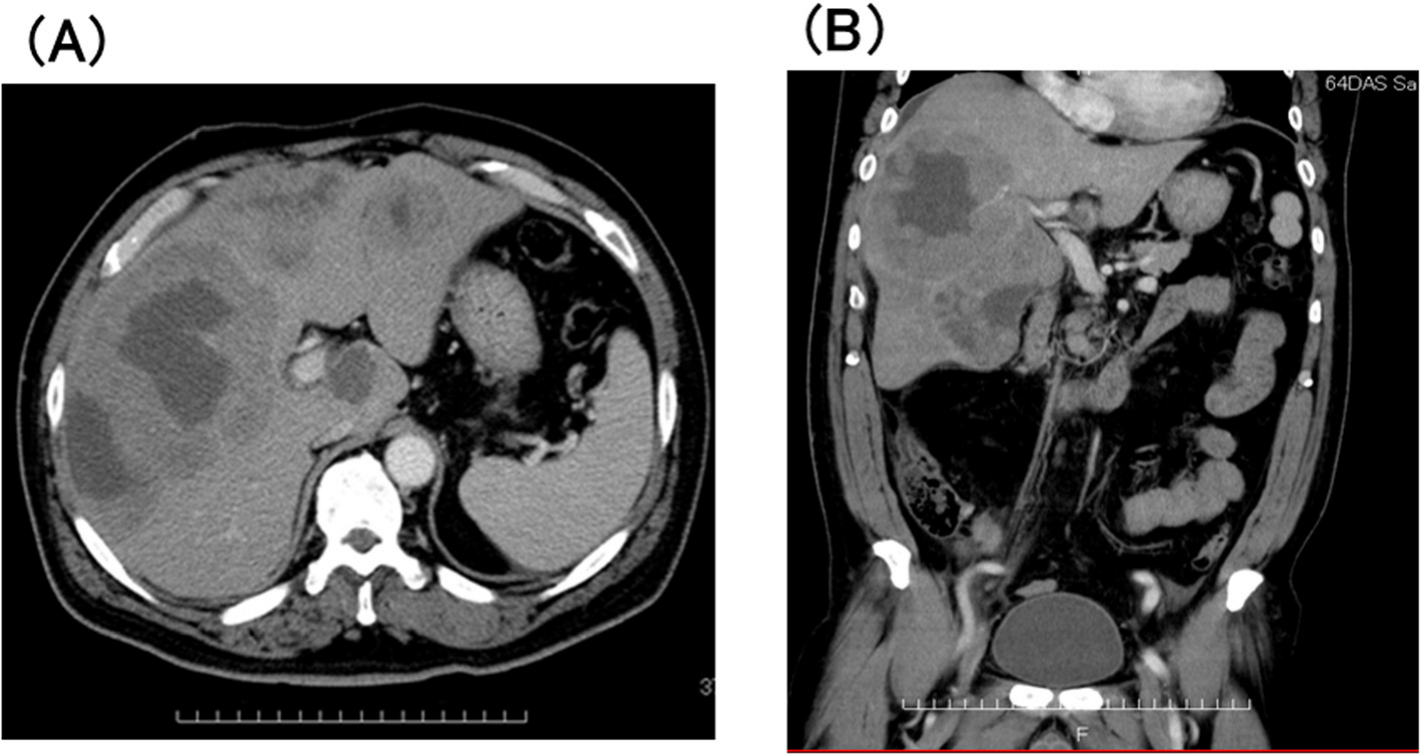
Contrast-enhanced computed tomography (CECT) of the abdomen at the time of admission (A) axial view; (B) coronal view showed multiple liver masses.
